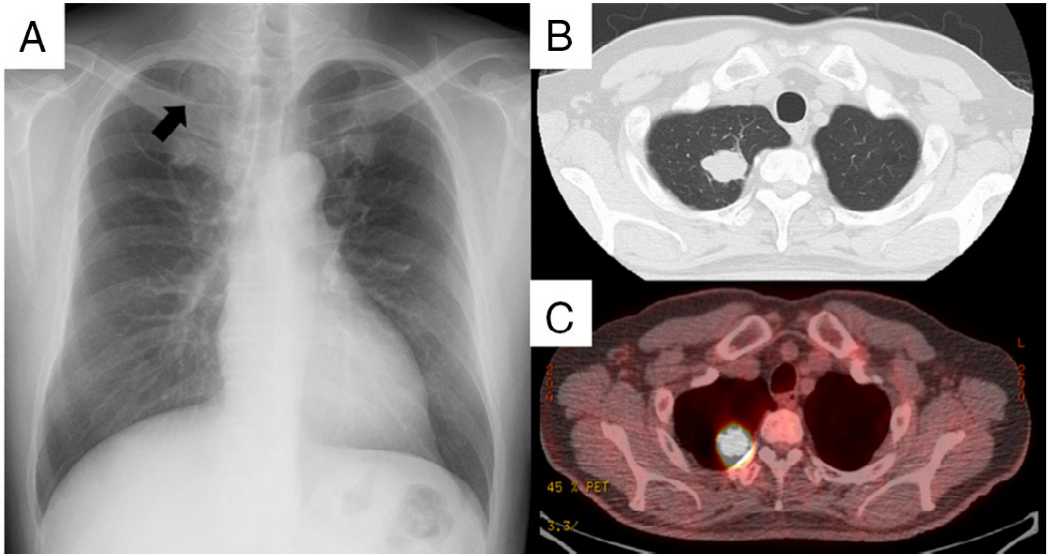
Chest X‐ray showing infiltration in the left upper lung field (arrow) at the time of referral (A). A solitary nodule with a size of 20 mm is seen on computed tomography (B). Intense uptake is shown in the lesion with 18F‐fluorodeoxyglucose positron emission tomography (C).
